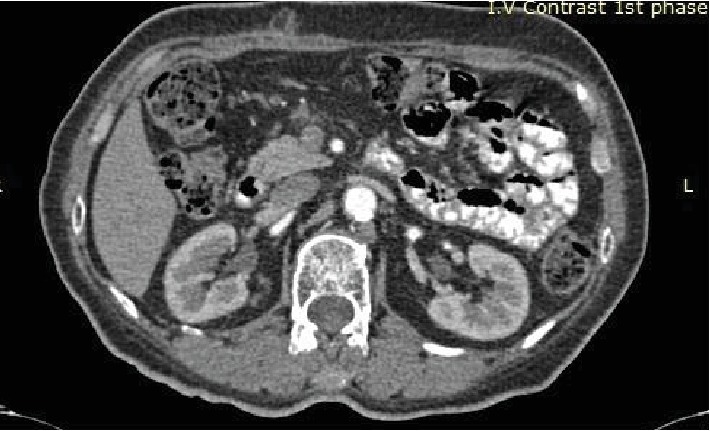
Follow-up computed tomography (CT image) with no evidence of recurrent disease.
